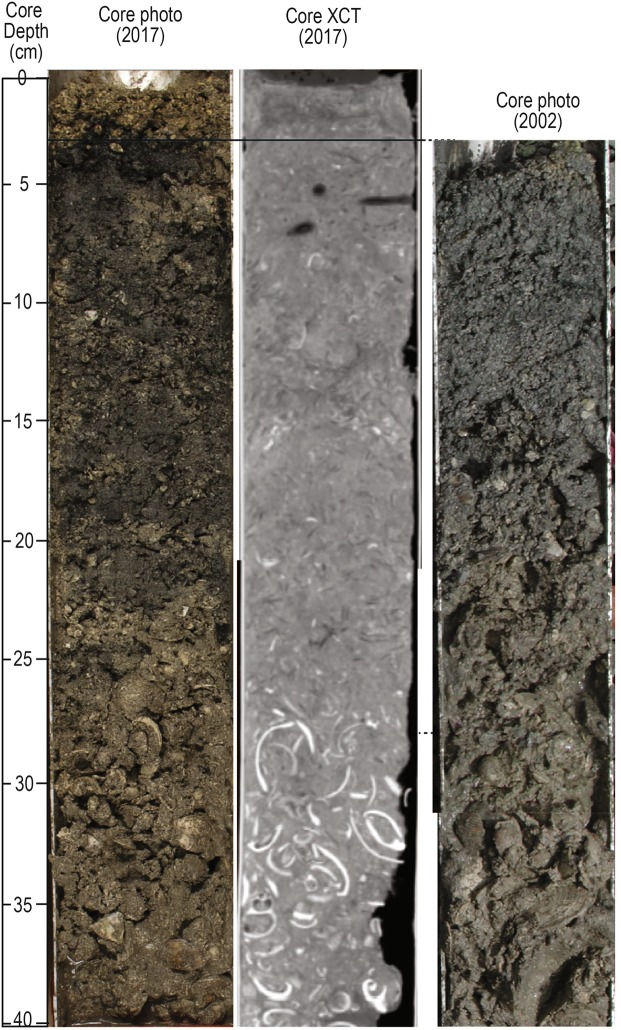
Core 2 collected in November 2017 (location in Fig. 4) showing a 3–4 cm thick surficial layer visible in the core photo (left) and XCT scan (middle). This layer was not present in a core taken in 2002 in the same location (right). This layer also contains Beryllium-7 indicating recent deposition (~months). X-Ray Computed Tomography (XCT).
